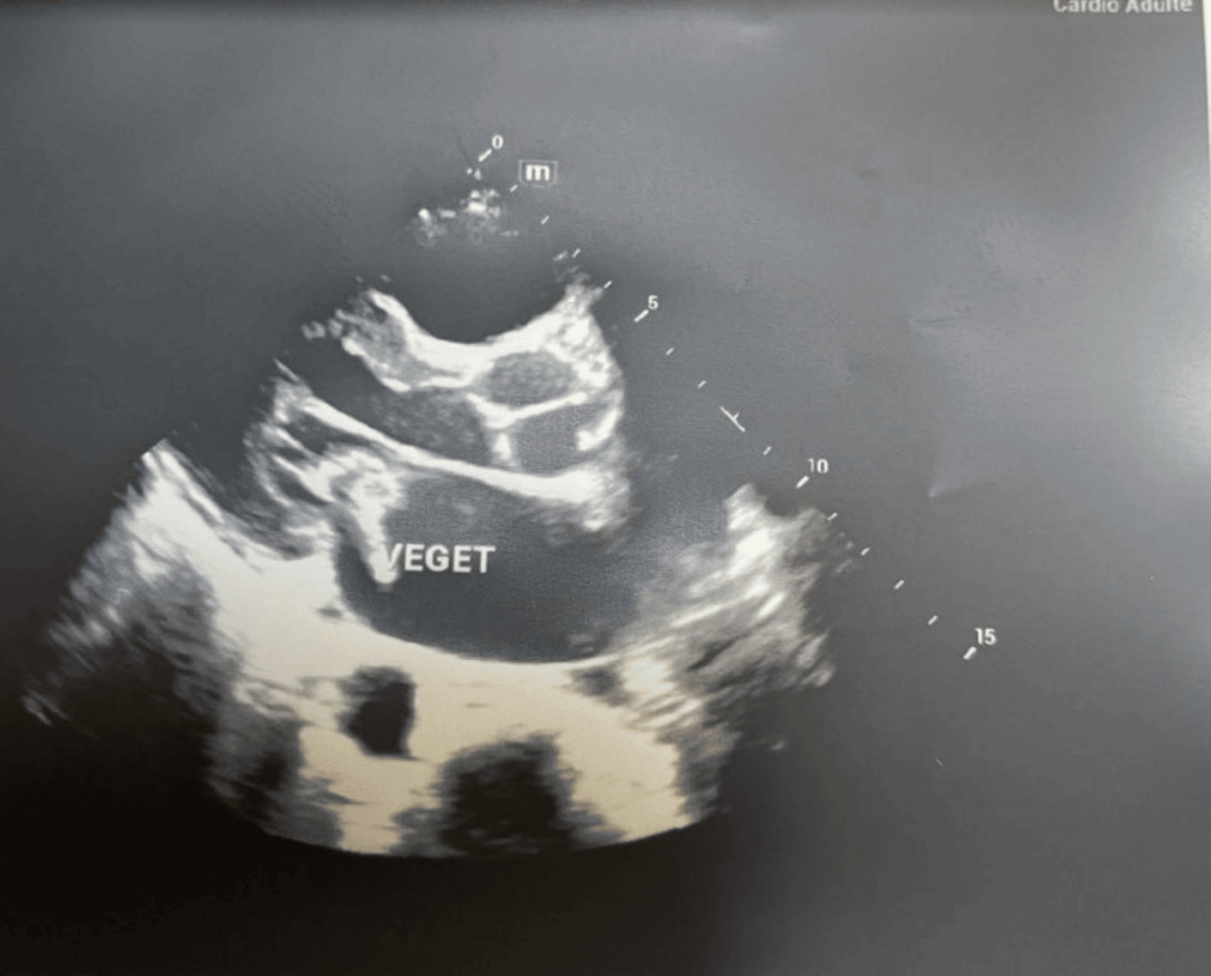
Transthoracic ultrasound image Transthoracic ultrasound image showing slightly thickened mitral valves, total restriction of the posterior valve, prolapse of A1, A2, and A3 associated with dilation of the mitral annulus responsible for severe eccentric leak, as well as the presence of a hyperechoic mass hanging on the atrial side of the anterior mitral valve, suspicious for vegetations.
